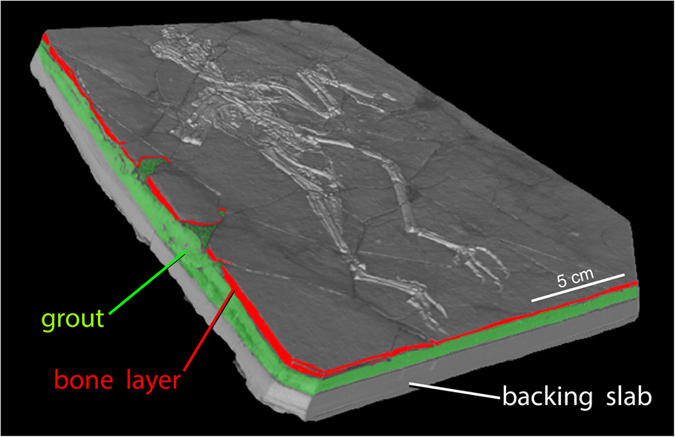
The *Confuciusornis* amalgamation, in oblique view. Volumetric rendering from CT data (Data Citation 1) showing its 3-layer man-made stratigraphy. The top layer is only 2–8 mm thick and was shattered during excavation. It was mosaicked together using a ceramic grout on a backing slab that provided structural integrity. Edges of the top bone-bearing layer are colored red; the middle layer consisting of grout is green; and the backing slab is gray.
